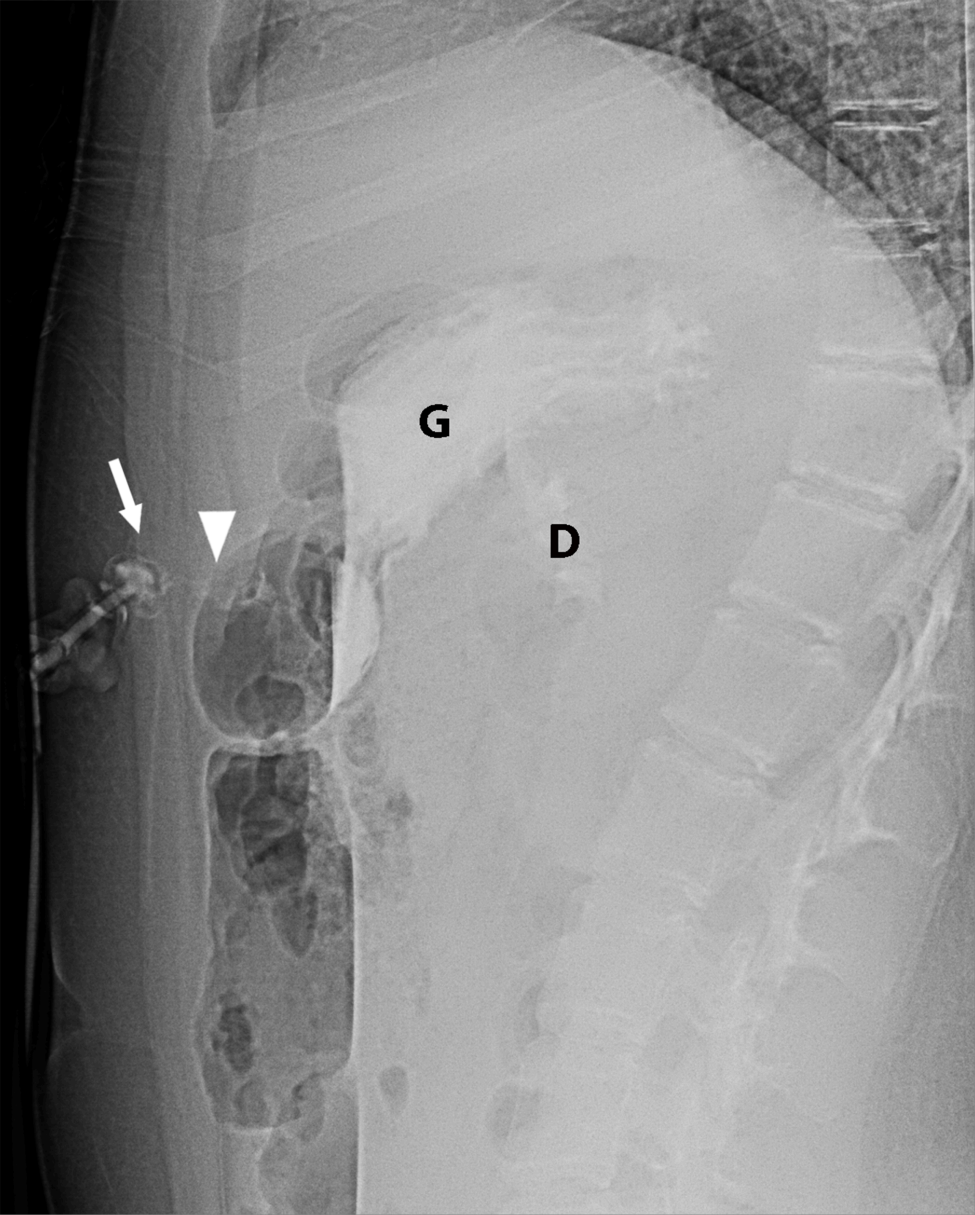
Cross-table abdominal radiograph shows gastric luminal contrast flow (G) and contrast in the proximal duodenum (D). However, the G-tube retention component (arrow) projects in the anterior abdominal wall in the superficial gastrostomy tract (arrowhead).
